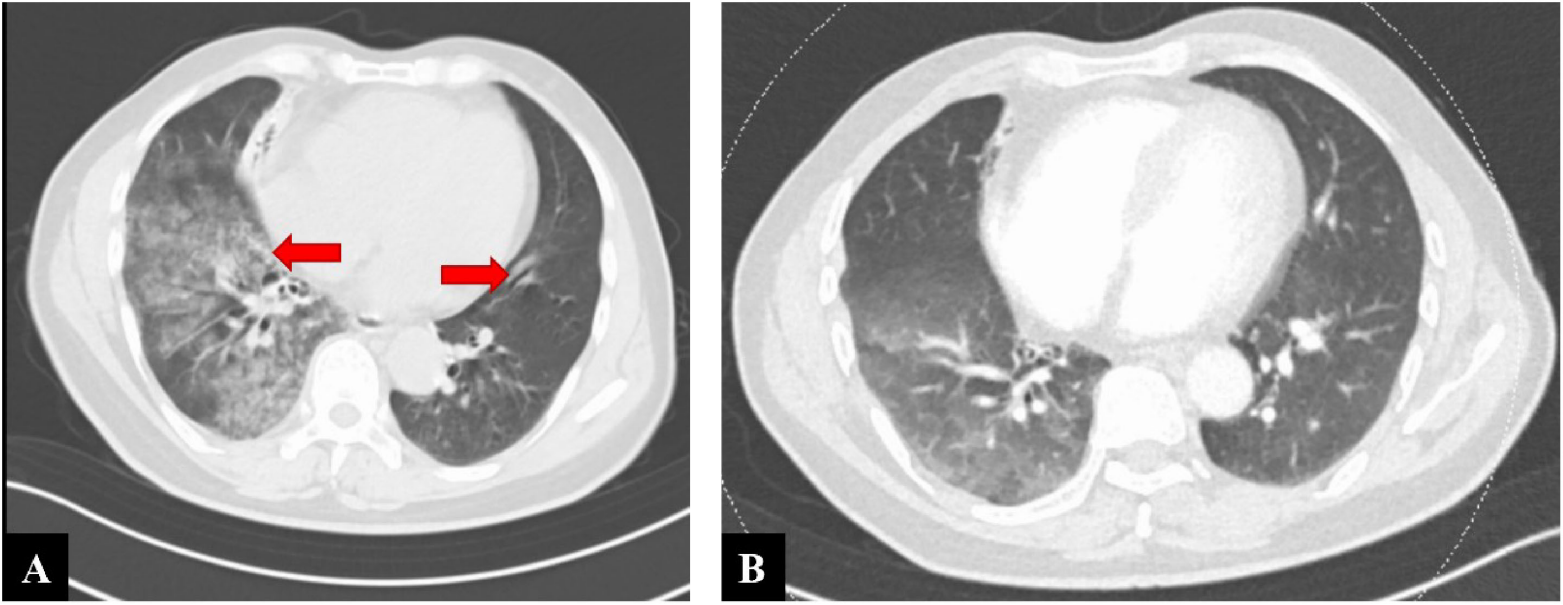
(A) Chest computed tomography (CT) on admission during massive hemoptysis, showing multiple ground-glass opacities in the lower lobes of both lungs (red arrows). (B) Follow-up chest CT after treatment, showing significant resolution of the opacities with no new hemorrhagic lesions.
